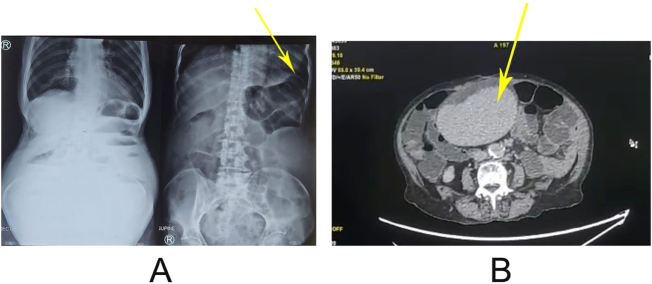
(A) X-rays shows dilated loops and (B) shows ectopic spleen on transverse section.
